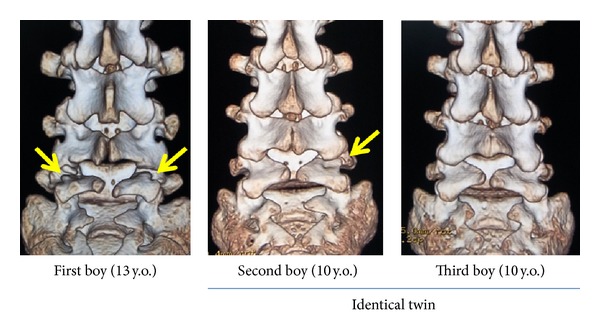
3D-CT of Cases  1–3, all showing similar structures.
